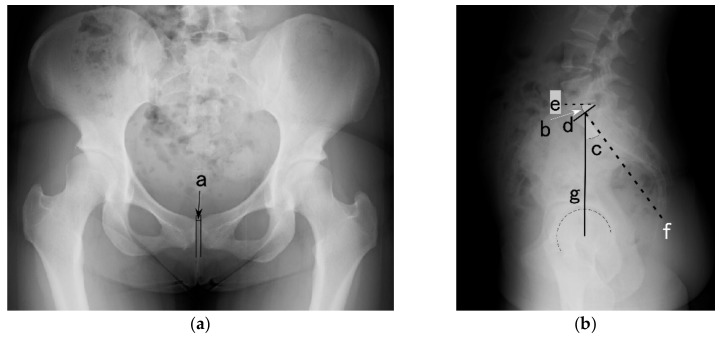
X-ray of the frontal image of pubic symphysis (a) and sagittal image of the lumbar region (b). a: pubic symphysis width, b: sacral slope, c: pelvic incidence, d: superior plate of sacrum, e: horizontal line, f: perpendicular line to the sacral plate at its midpoint, g: line connecting the midpoint of the sacral plate to the center of the femoral head.
